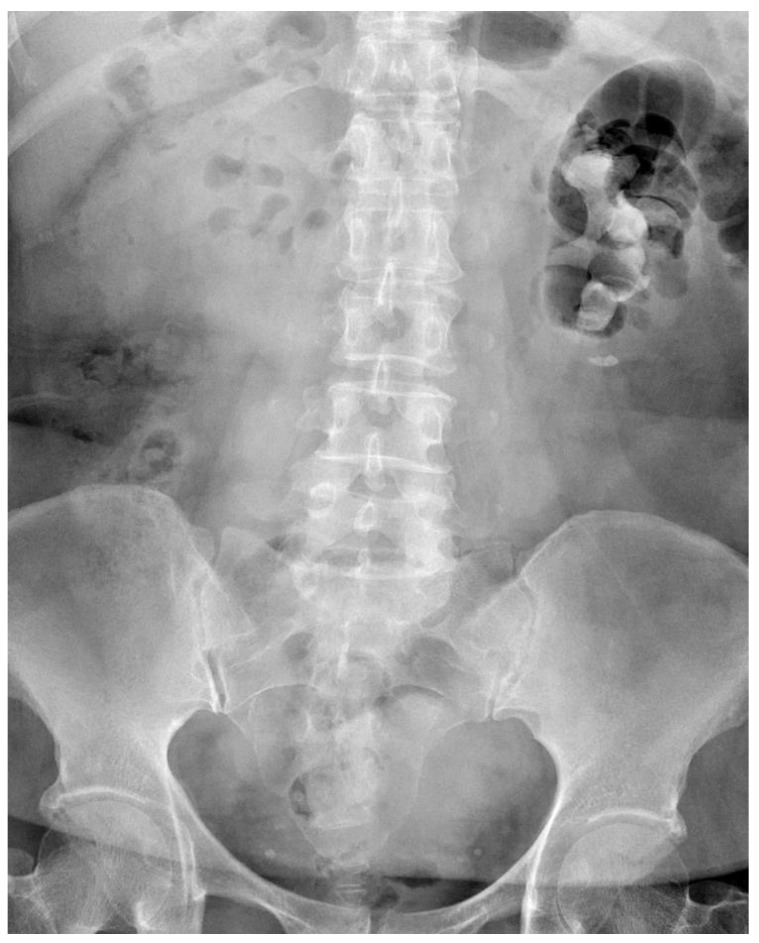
Preoperative findings of a kidney, ureter and bladder radiograph that were suggestive of a large staghorn stone about 7 cm in size.
